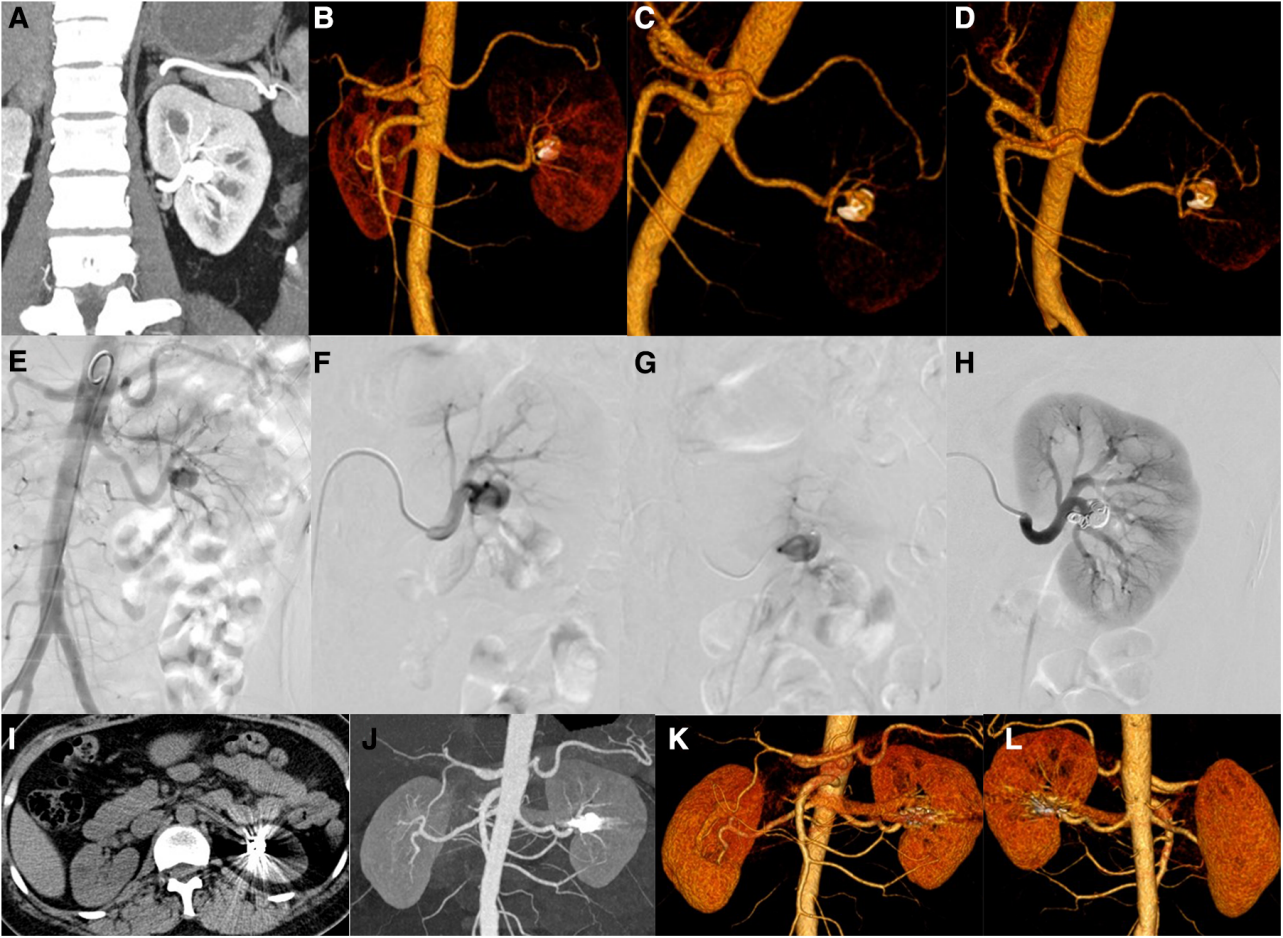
Patients no. 1 with renal aneurysms: (A–D) Computed tomography (CT) showed an aneurysm (1.4 cm × 1.2 cm) at the proximal end of the posterior segment of the left renal artery, accompanied by mural thrombus and peritumoral calcification, and several cysts in the right kidney and liver. (E,F) An angiography of the left renal artery is performed, and frontal imaging (digital subtraction angiography) showed that the left renal artery was equivalent to the artery adjacent to the left renal pelvis and showed a tumor-like expansion (approximately 1.1 × 1.4 cm). The left renal artery aneurysm is embolized with a microcoil and medical glue through a microcatheter, and the left renal artery angiography is repeated. (I–L) Three months after the operation, the CT scan showed that the posterior segment of the left renal artery showed a metallic fixation, which showed post-embolization changes.
